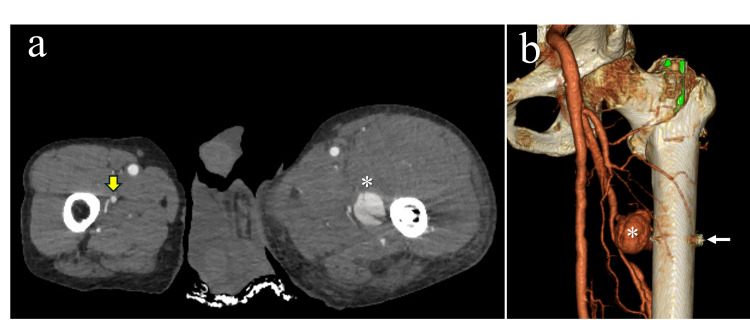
Postoperative contrast-enhanced computed tomography DFA: deep femoral artery (a) Axial image at the level of distal screw insertion. The affected thigh is swollen to approximately 1.5 times the transverse diameter of the contralateral side. A pseudoaneurysm with a false lumen is observed in the DFA (asterisk). The contralateral DFA lies 8 mm medial to the femur (thick yellow arrow). (b) Three-dimensional reconstruction showing a pseudoaneurysm (asterisk) at the same level as the distal screw insertion site (thin white arrow)
